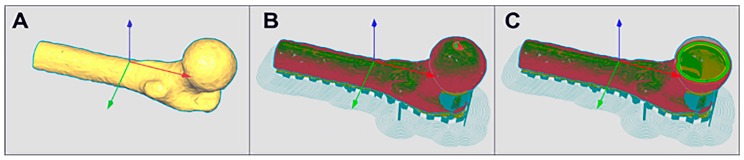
(A) The 3D femur model reconstructed from the CT images of a deidentified real patient. (B) The hollow mold model which was used to 3D print the Polyvinyl Alcohol (PVA) mold. (C) demonstrates the hollow interior space within the reconstructed model shown (A). The arrows indicate the X, Y and Z axes.
